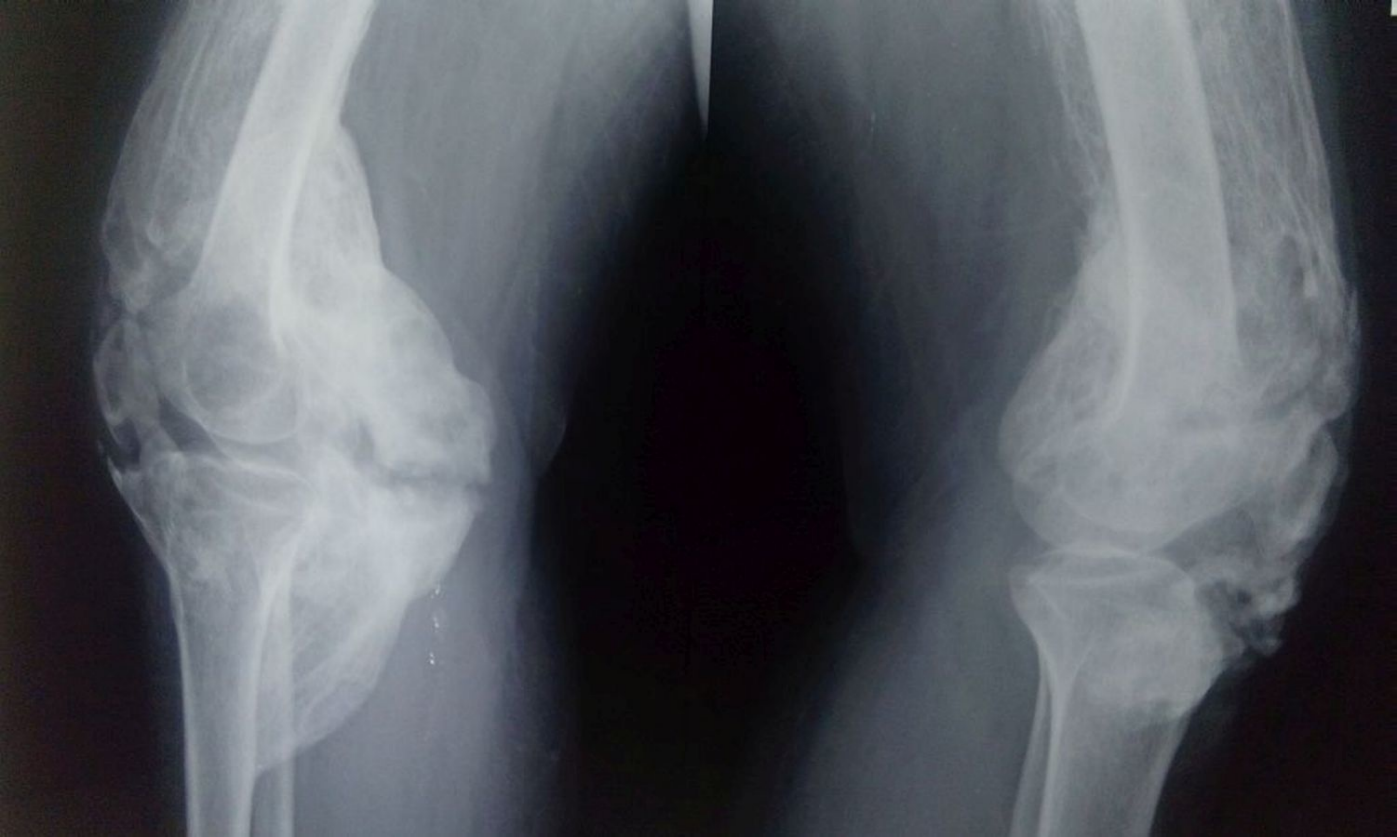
Lateral radiographs of the knees showing circumferential presence of diffuse heterotopic ossification
